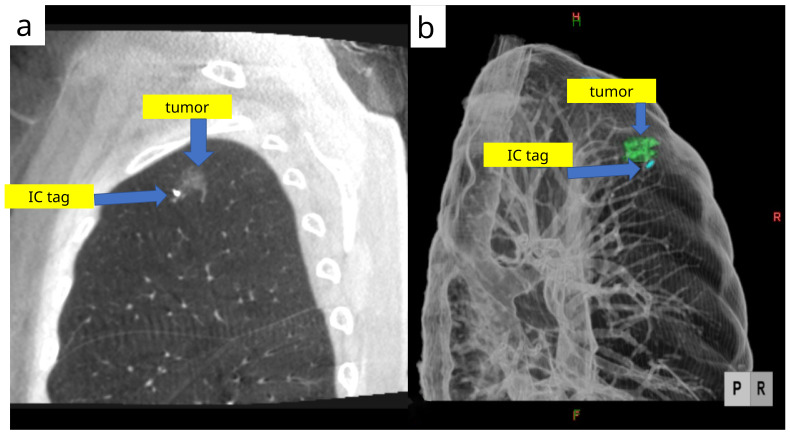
Cone-beam CT images after delivering an RFID (SuReFInD) tag: (a) sagittal section; (b) 3D reconstructed image. Source: original institutional cone-beam CT image; included to demonstrate postdeployment confirmation of an RFID tag relative to lung anatomy and to clarify the depth-aware nature of this localization strategy.
